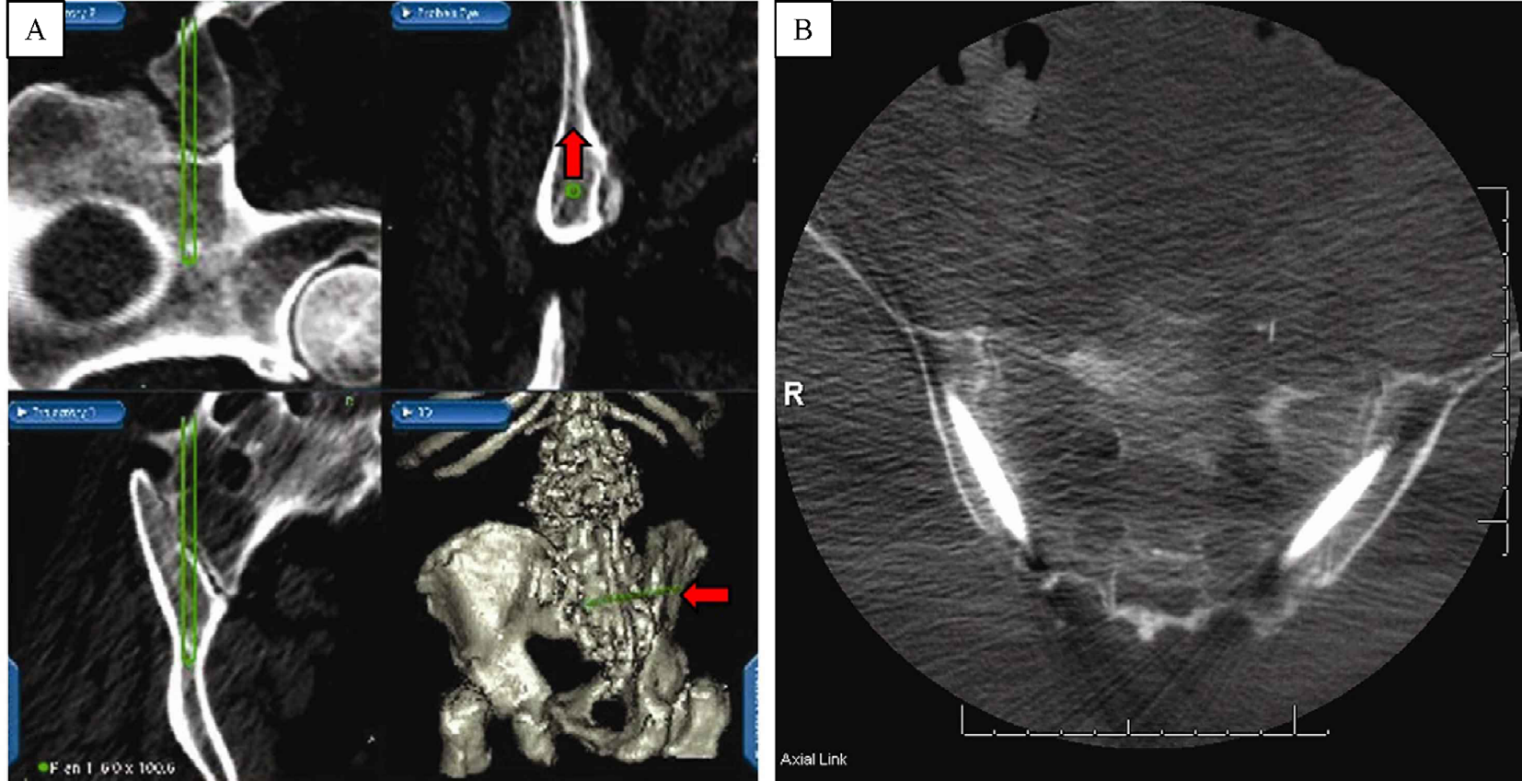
Stealth IGS monitor snapshot. A: Upper left: parasagittal view showing the trajectory from the sacrum to the pelvis. Upper right: probe’s eye view of the ilium. This view is particularly helpful for planning a screw trajectory that avoids cortical breech and has an ideal location, where a screw may be surrounded by stronger cortical bone (arrow). Lower left: the axial view of sacropelvic screw trajectory. Lower right: 3D view of the sacropelvic region. Note the extensive iliac defect (arrow) on the right from an iliac crest harvest performed during previous surgery. Image guidance is helpful in planning the trajectory to avoid the area of defect/weakness. B: Postoperative axial CT showing good placement of bilateral sacroiliac screws.
